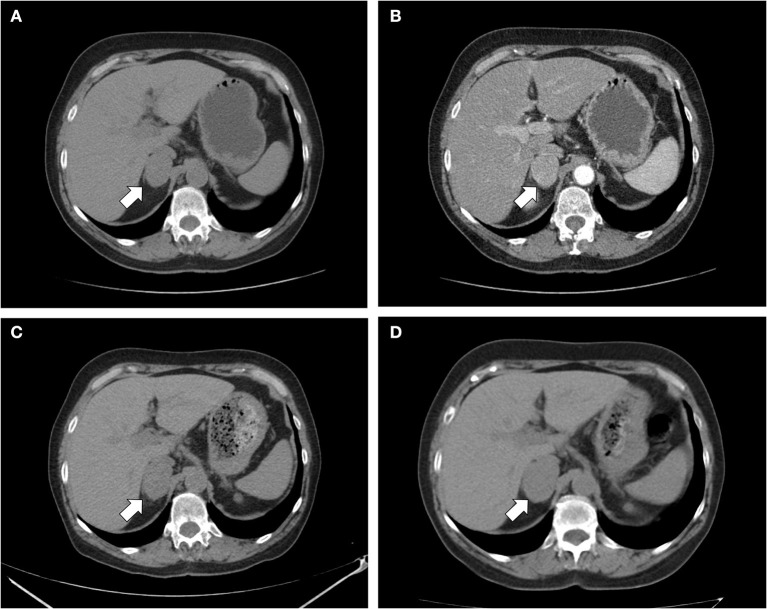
CT scan of the abdomen. (A) Unenhanced and (B) contrast-enhanced CT scan performed at diagnosis showed a homogeneous right adrenal mass of 32 × 40 mm with density of 35 HU and relative contrast washout of 21% (white arrow); (C) after 8 months, the CT scan showed a substantial stability of the adrenal lesion; (D) after 24 months, a slight dimensional growth of adrenal nodule (32 × 44 mm) and change in its density (39 HU) was found.
